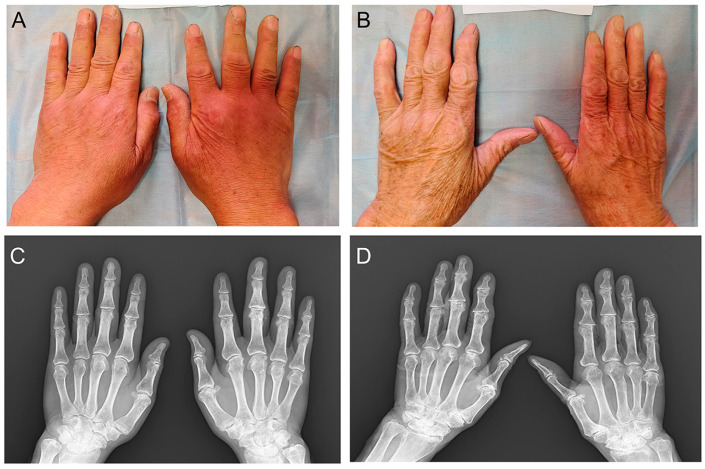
Characteristics of the study samples with Kashin–Beck disease. (A) Hand images of male patient; (B) hand images of female patient; (C) hand X-ray of male patient; and (D) hand X-ray of female patient. The image (A) and X-ray (C) are from the same patient, while the image (B) and X-ray (D) are from the same patient.
